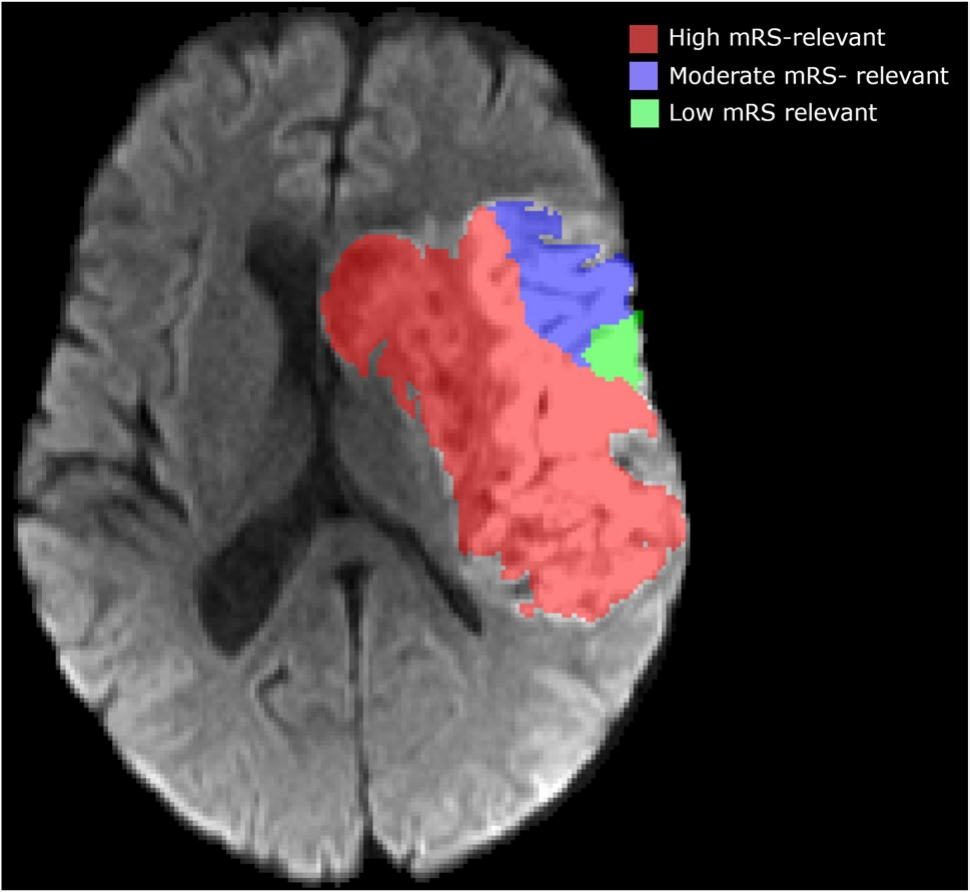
An example of the infarct segmentations on diffusion weighted MRI in areas with different mRS relevance
